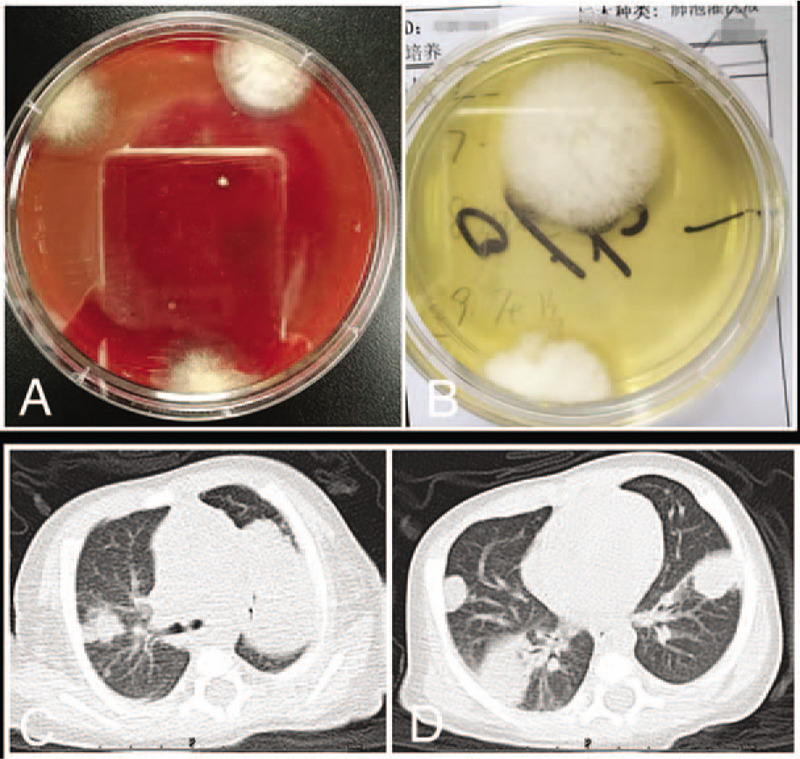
Patient #1, a 34-day-old boy admitted to the hospital for cough for 1 week, and fever for 1 day. (A) BALF culture for bacteria showed the growth of *Aspergillus fumigatus*. (B) BALF culture for fungi showed the growth of *A fumigatus*. Thoracic computed tomography at admission (C-D) showed multiple nodular high-density shadows or mass-like shadows.
